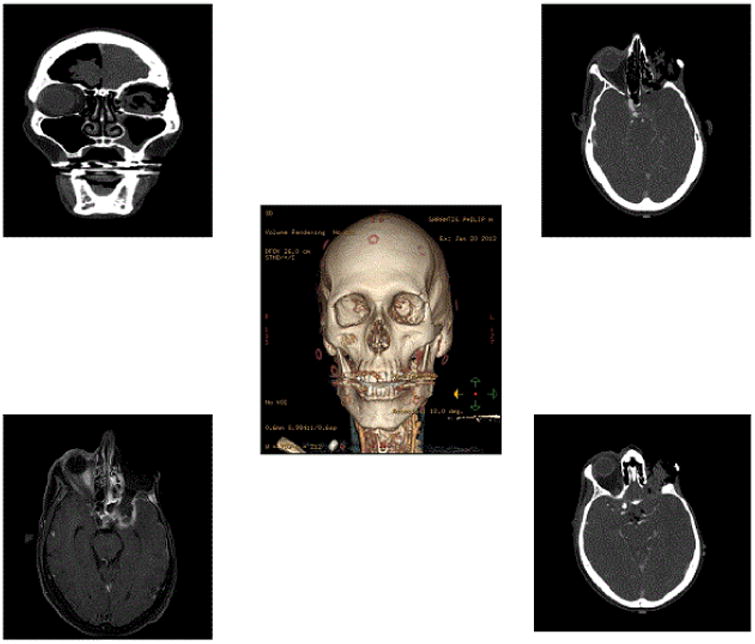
Pre-operative CT imaging showing pneumocephalus and signs of meningitis
